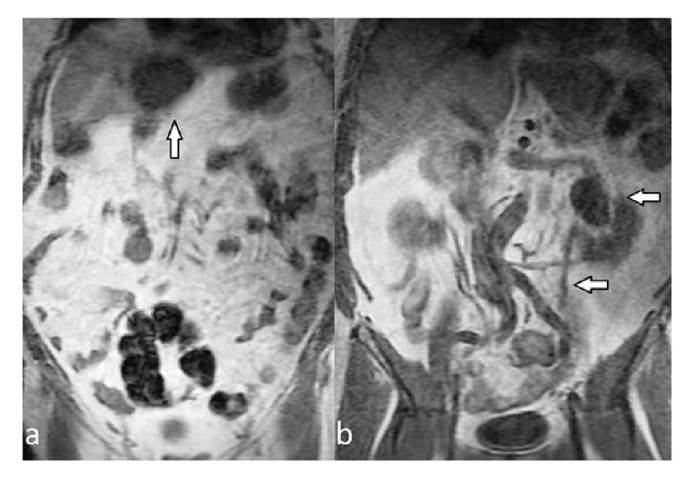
A hypointense mass (arrow) is observed in the liver (a). Coronal T1-weighted images show pelvic dilatation of the left kidney (arrows). There is also dilatation of the left ureter (b).
